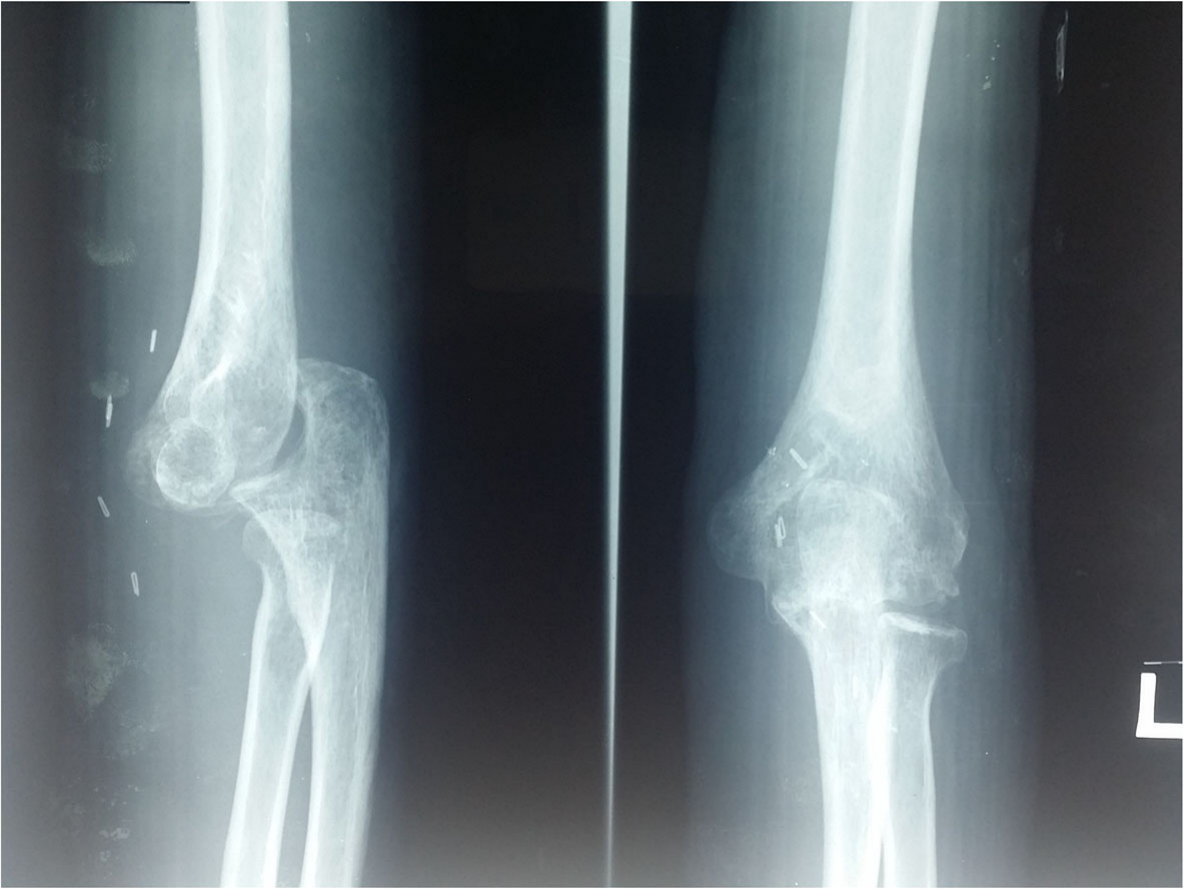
Radiograph of left elbow after closed manipulative reduction
